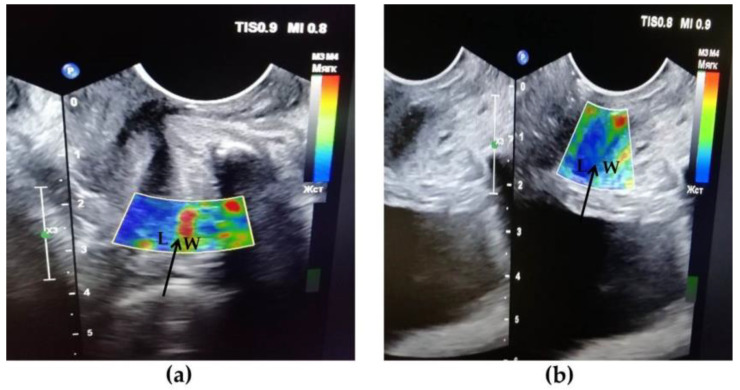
Compression ultrasound (US) in the normal condition (a) and in UPS (b). (a) Normally, the urethral wall is softer (red color) than in UPS (b) (predominance of green and blue colors). L—lumen of the urethra, W—urethral wall. The black arrow indicates the border between the lumen of the urethra and its wall.
